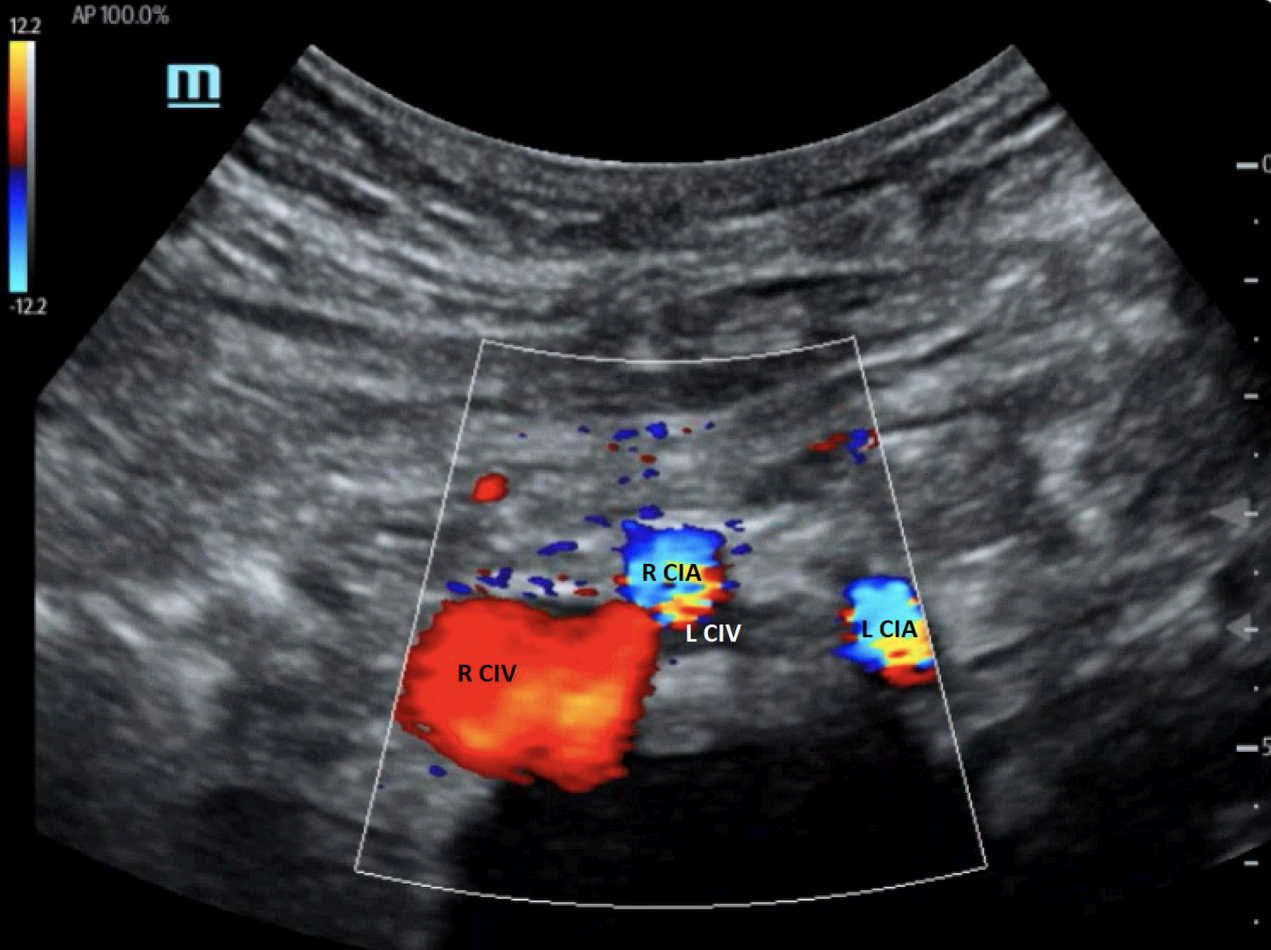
Color Doppler image of iliac vein compression syndrome. The left common iliac vein (L CIV) is compressed by the right common iliac artery (R CIA). R CIV, right common iliac vein; L CIA, left common iliac artery [From: Brown et al. (39). Reprint under a Creative Commons Attribution 4.0 International License].
